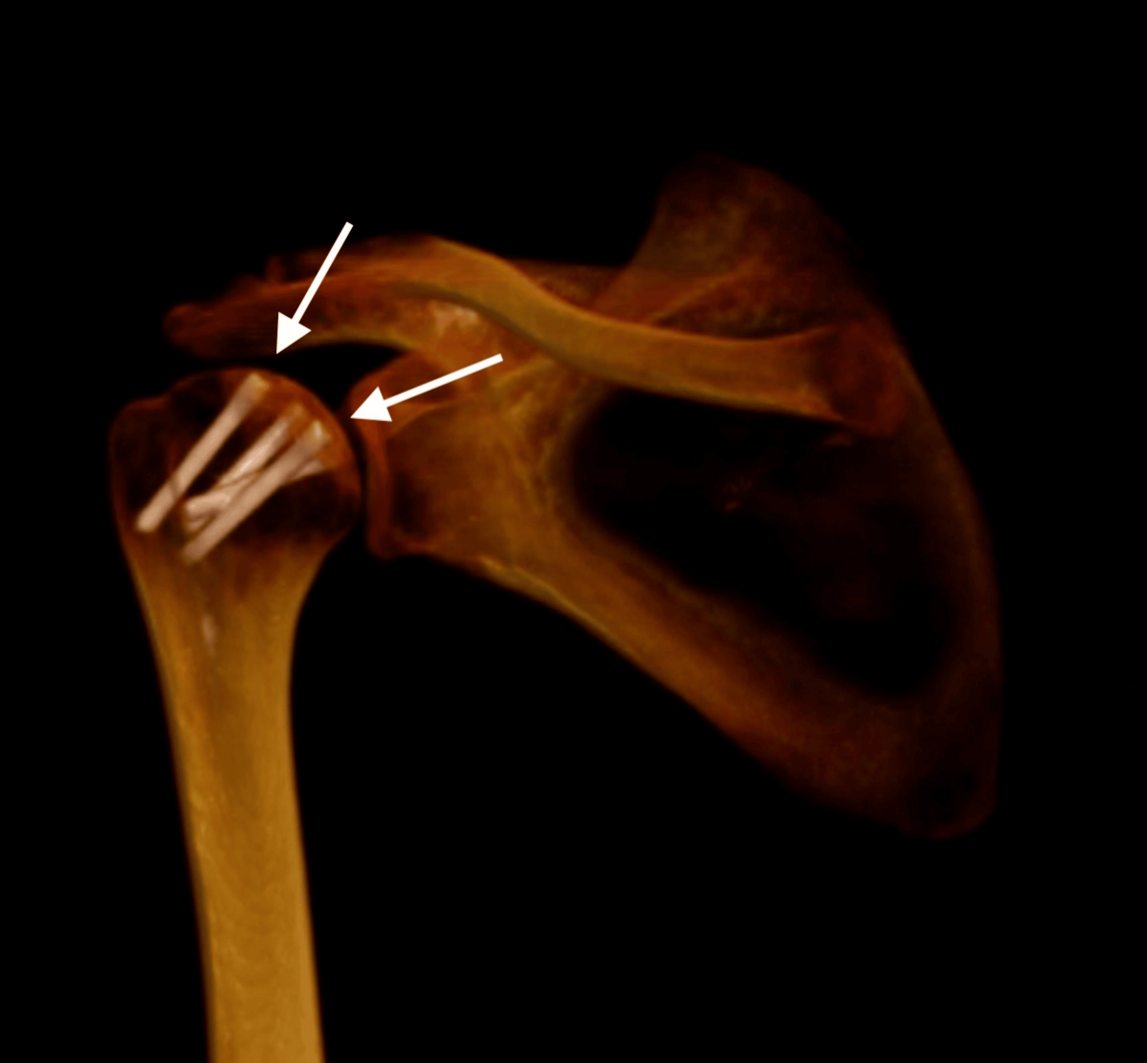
A 3D metal suppression CT reconstruction at the one-year follow-up visit The white arrows signal adequate subchondral localization of the screws.
